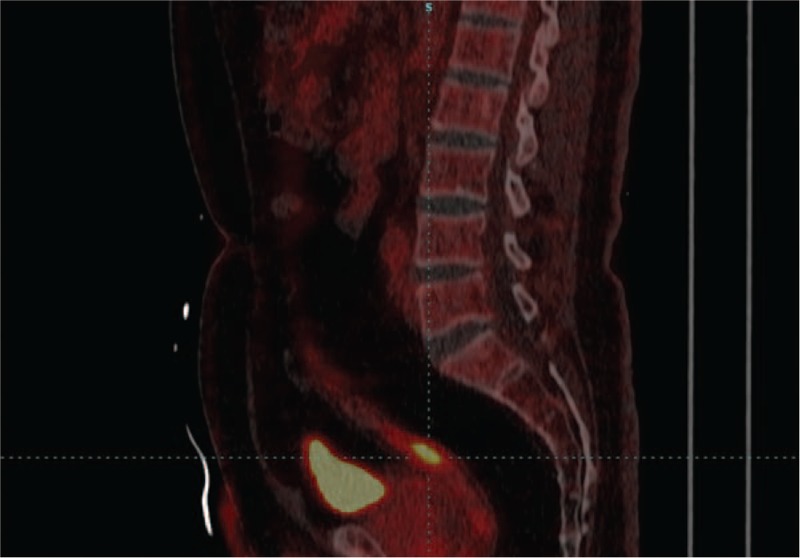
Sagittal view of FDG-PET/CT: abnormal increased radioactive uptake in the upper rectum.
